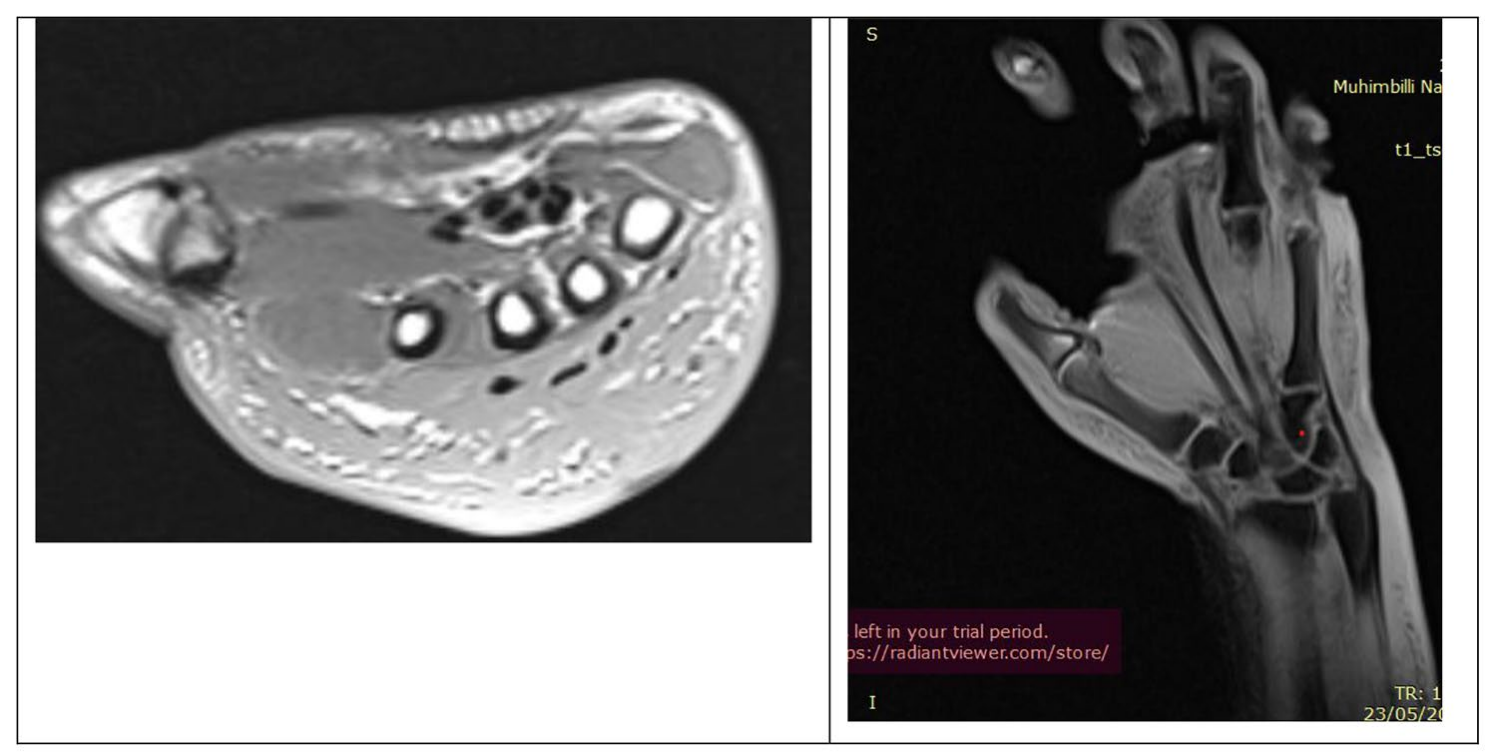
MRI images of the hand: axial and coronal cuts
